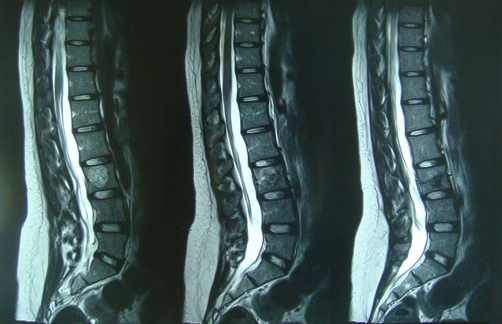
Preoperative imaging. MRI of the patient with L_3_ hemangioma. Note a silent fracture that was missed preoperatively.
